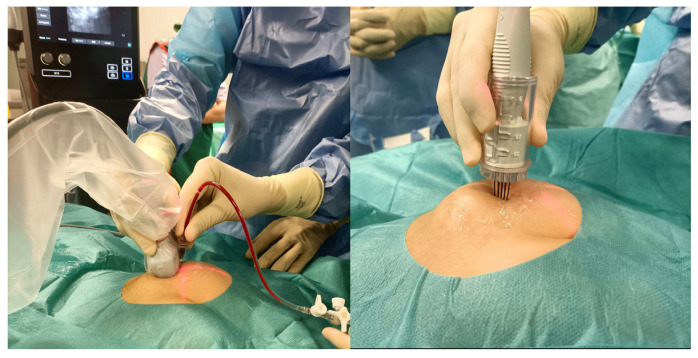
Intraoperative view of the intralesional injection of a solution of bleomycin and contrast agent under ultrasonographic guidance (left), follows by the application of electrical pulses generated by the electroporation device (right).
